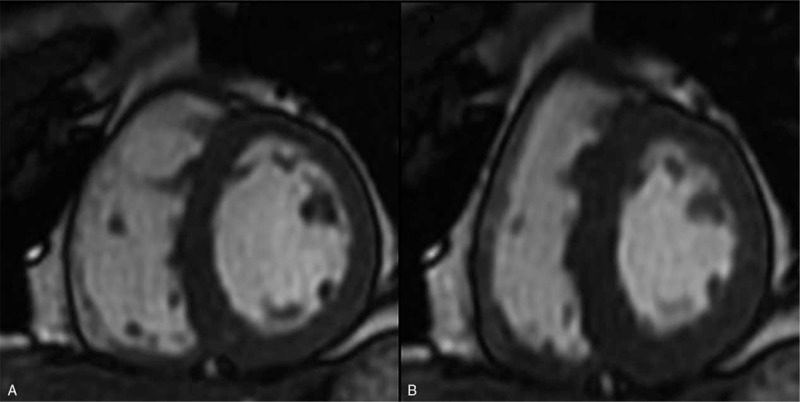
(A repeated MRI after 17 months): Cine images during end-diastolic (A) and end-systolic (B) phases demonstrated global normal ventricular wall thickness (left ventricular wall thickness, including septal wall, was 8–12 mm, and right ventricular wall thickness was 4–5 mm) in the short axis at the level of the central ventricles. The pericardial effusion was absorbed completely, with a normal left ventrical ejection fraction of 79%. ^∗^MRI = magnetic resonance imaging.
